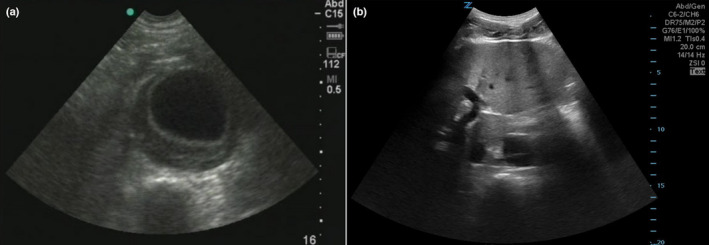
Ultrasound images of aortic aneurysm (a) and aortic dissection (b) in transverse using a low‐frequency curvilinear probe. [Colour figure can be viewed at wileyonlinelibrary.com]
